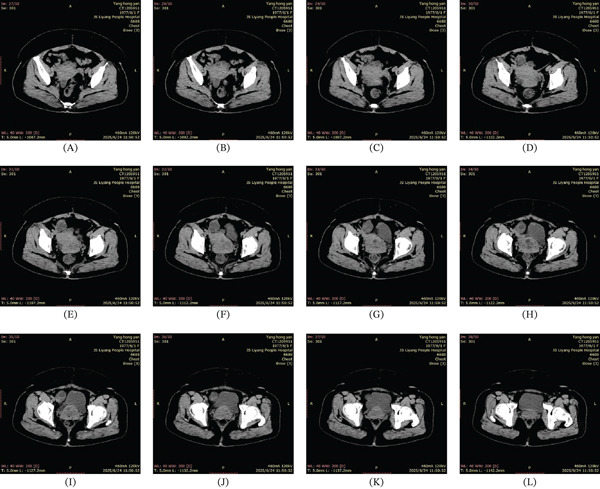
(A–L) Preoperative computed tomography (CT) features of a Müllerian duct remnants on the uterine surface with ovarian serous cystadenoma.
